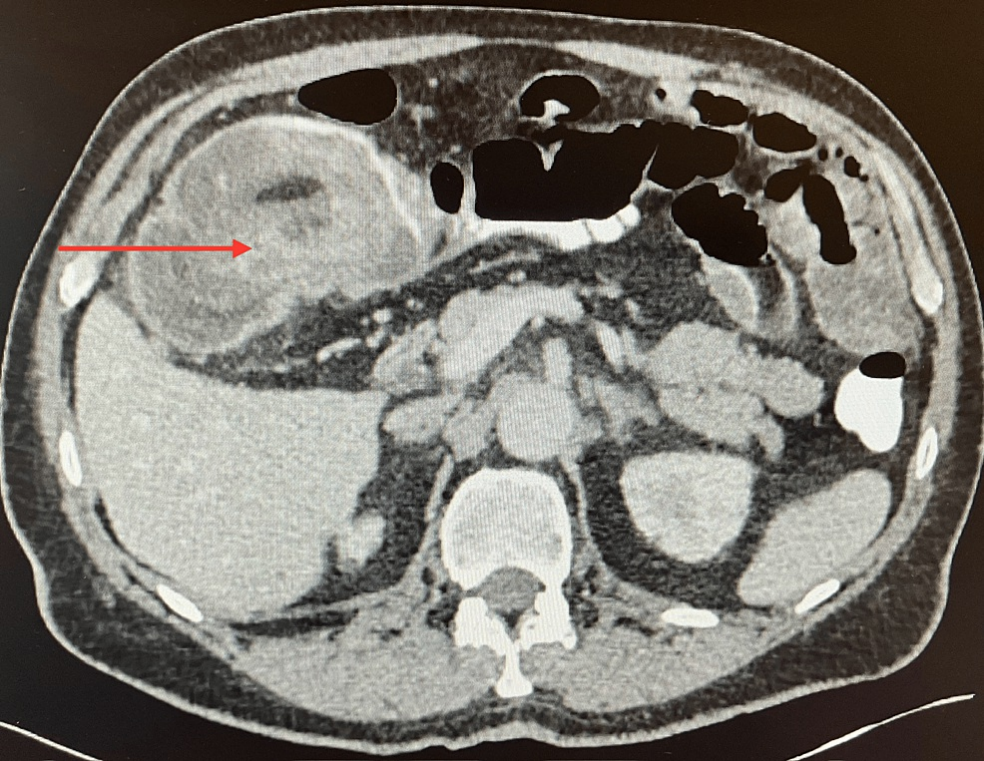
CT abdomen (axial) showing right colon intussusception (red arrow)
